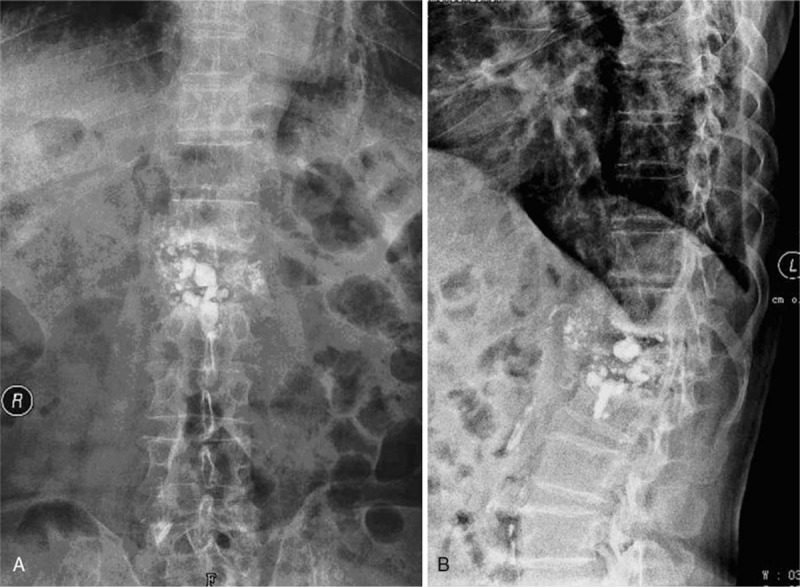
Plain radiograph showing L1 vertebral collapse and PMMA breakage, with a kyphosis angle of 25° (March 2016). PMMA = polymethylmethacrylate.
